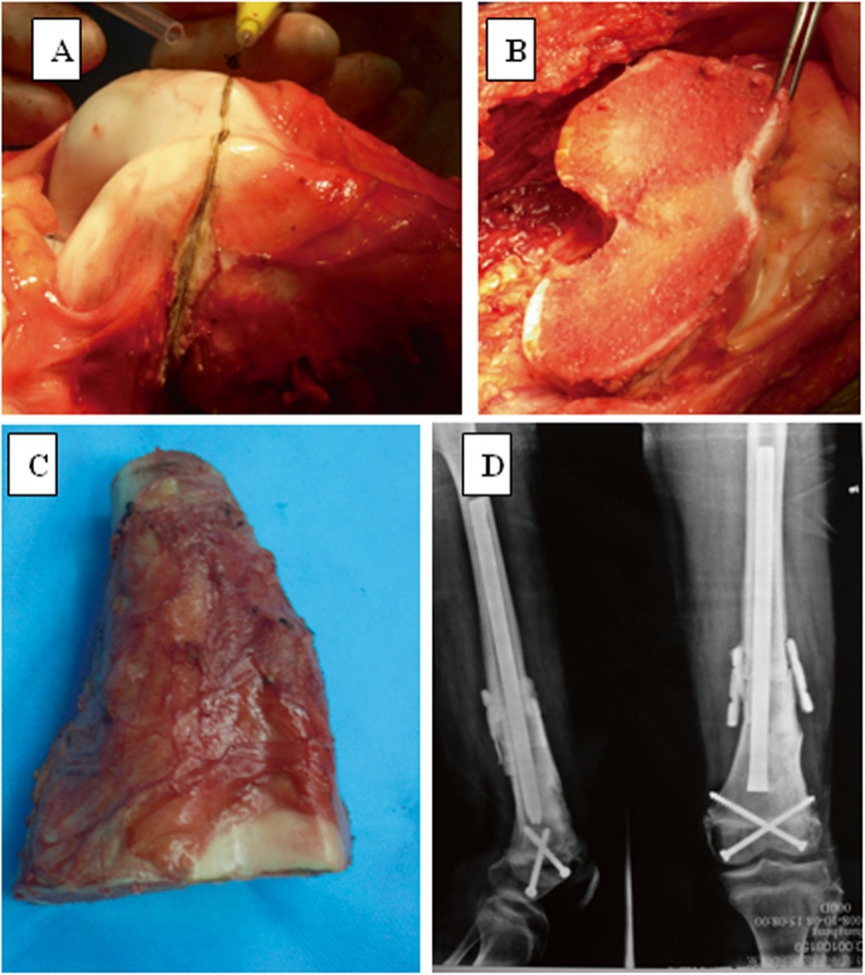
Results for a patient treated for osteosarcoma. (A) The epiphysis of the distal femur was preserved by marginal resection; (B) the remaining epiphysis; and (C) the resected tumor. (D) The radiograph shows that after an intramedullary nail and screws had been inserted into the residual epiphysis and the bone defect filled with bone cement (no barium), the implanted bone healed successfully.
